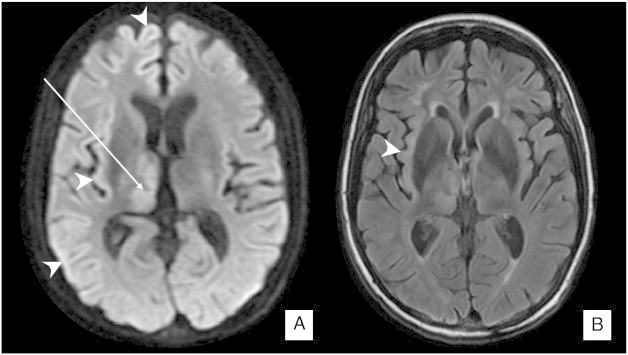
A. DWI obtained 5 days after admission reveals a subtle, increased signal over the large area of the right cortical ribbon, most prominent over the hippocampus (arrow). Right thalamic involvement is more prominent (arrow). The ADC map reveals a subtle low signal consistent with cytotoxic edema (not shown). B. FLAIR sequence on the same day reveals a higher signal mainly over the right hippocampus and ipsilateral thalamus.
